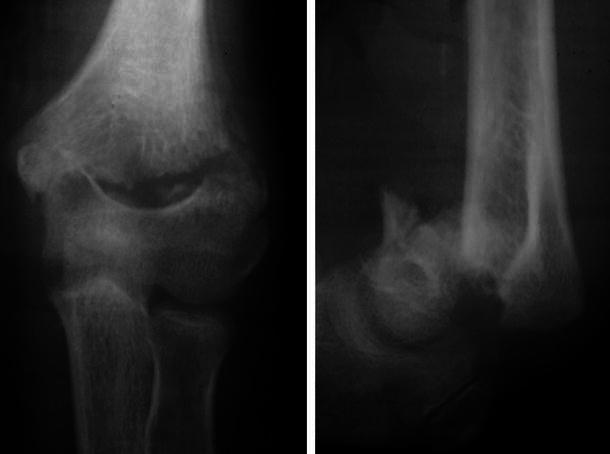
X-ray of a patient with flexion-type supracondylar fracture humerus neglected for 22 days showing off-ending of the fragments with marked anterior displacement of the distal stump with early callus formation (seen in antero-posterior view) (patient 14)
